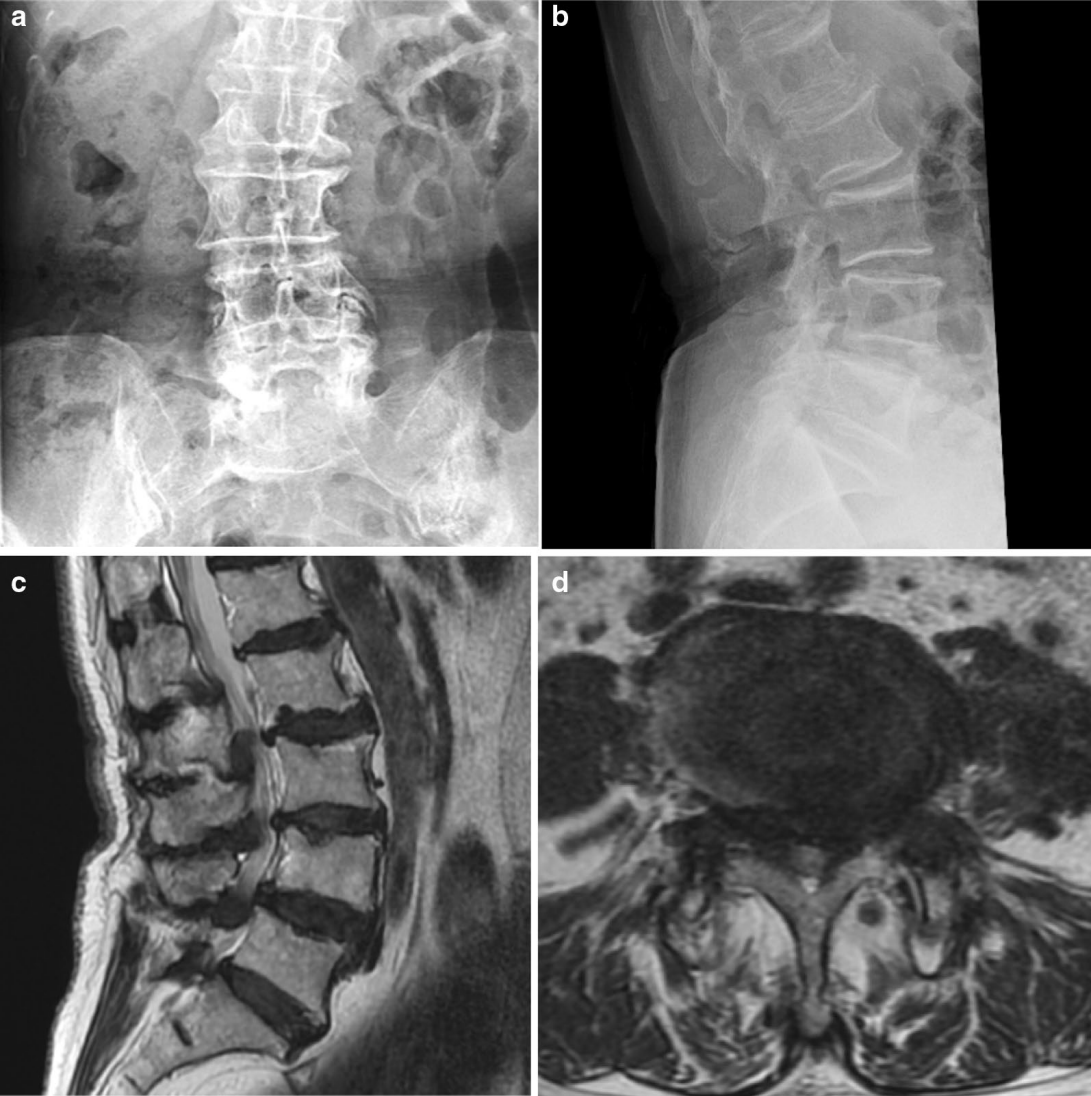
Preoperative radiography (a, b) and magnetic resonance imaging (c, d) revealed L4/5 lumbar spinal stenosis
